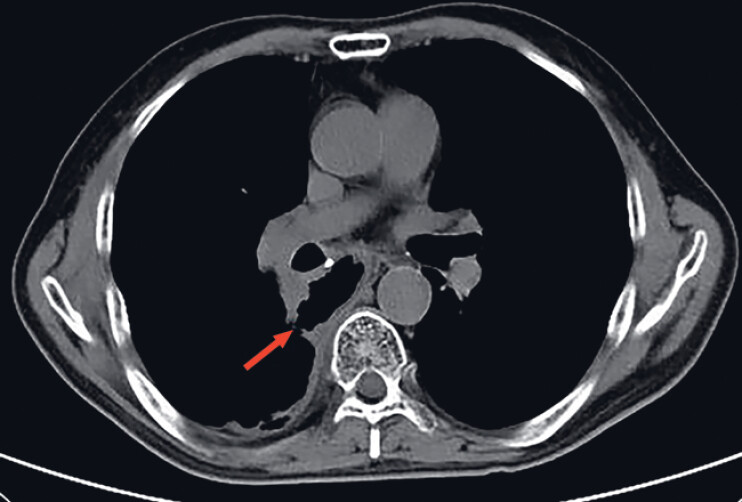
Thoracic computed tomography image showing a right-sided gastrobronchial fistula.
